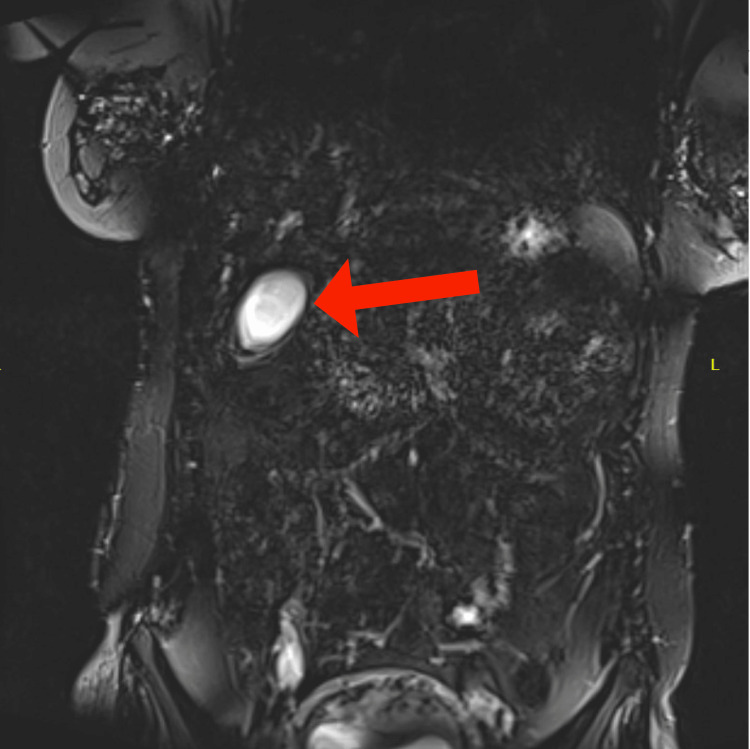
Normal abdominal MRCP of the patient. Coronal T2-weighted image from MRCP of the abdomen with normal gallbladder appearance (red arrow). MRCP: magnetic resonance cholangiopancreatography
